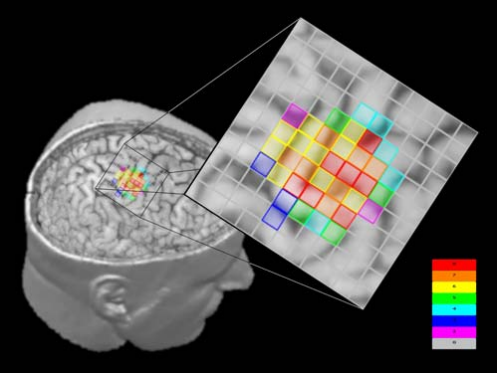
TMS-MRI integration: number of co-activated muscles for each scalp position in a paradigmatic subject.
